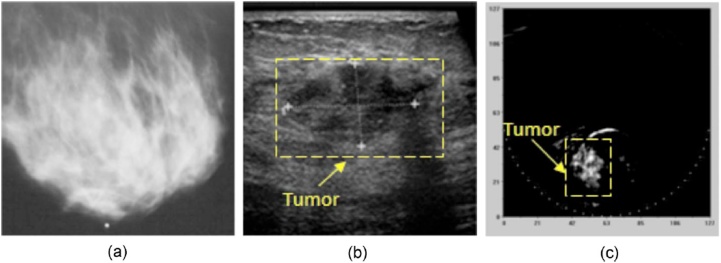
Using the LOIS-64 – semi-circular aperture imager: a case of breast with an infiltrating ductal carcinoma. (A) The lesion is occult in the radiographically dense breast in the X-ray image. (B) The US image reveals the tumor at 21 mm deep. (C) The LOIS image reveals the lesion with strong contrast, pointing to vascularization which is indicative of a malignant tumor. (Reproduced with permission of the authors and publisher.)
